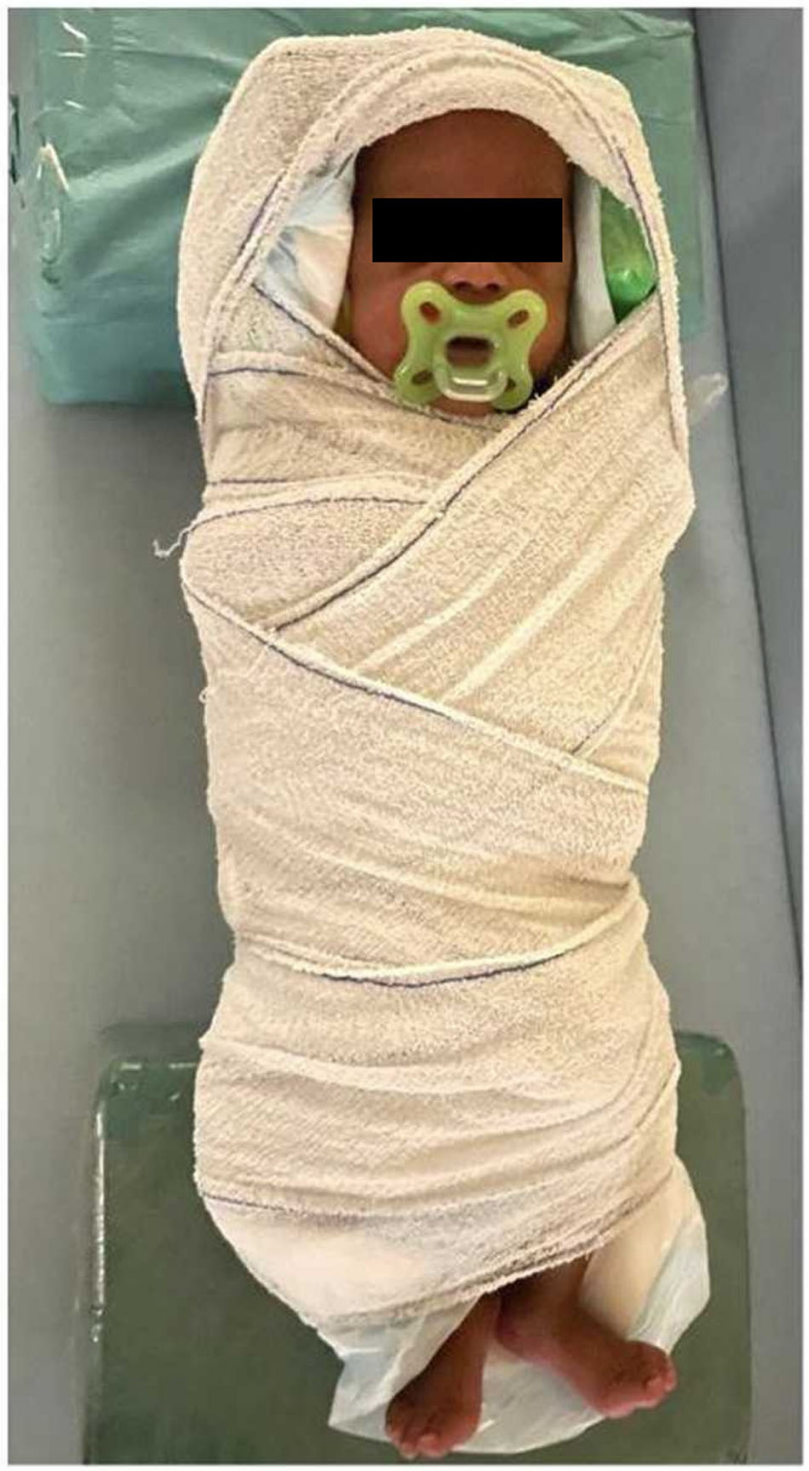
Immobilized infant for MRI. Note how the ear protection equipment is tightly secured on the ears by the wrap. Figure adapted from Korom et al [32], released under a CC-BY license
